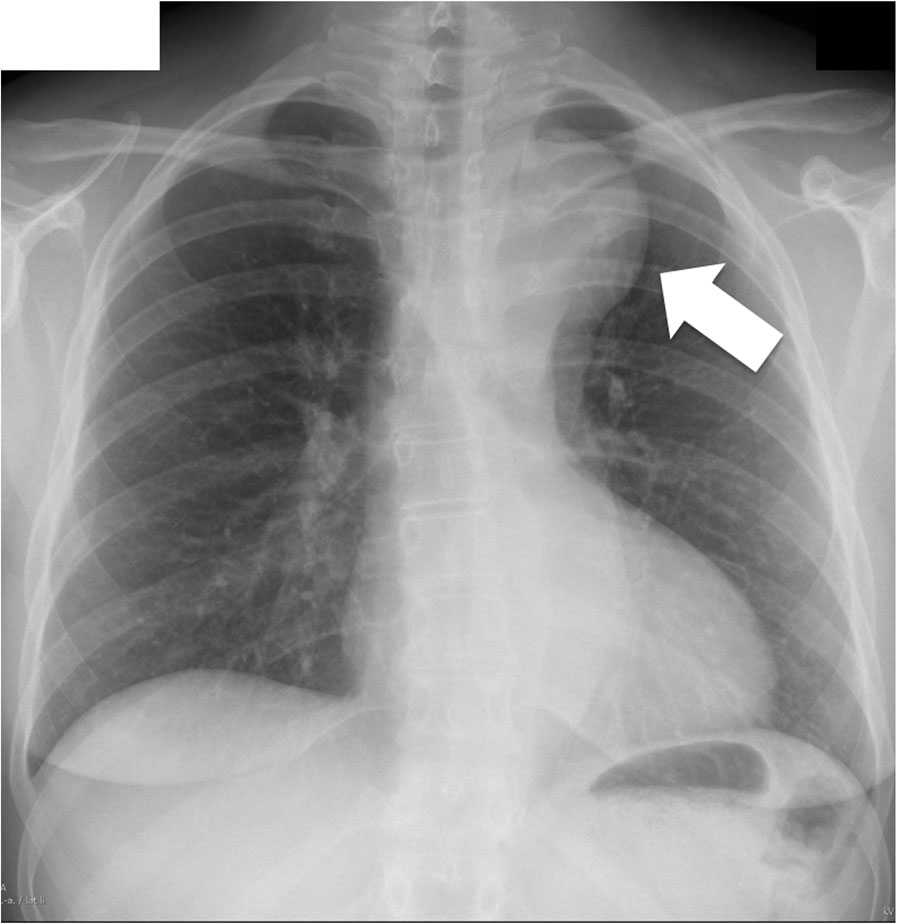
Chest-x-ray with enlarged mediastinum (arrow)
